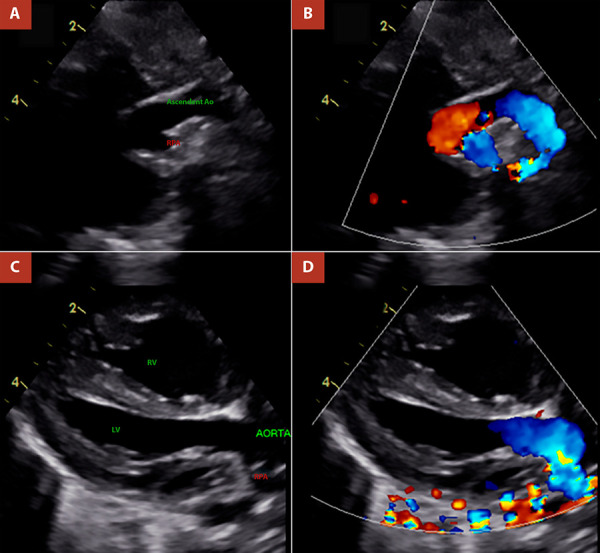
Transthoracic echocardiography, Case 02. A) and B) Suprasternal views showing the origin of the right pulmonary artery from the posterior wall of the ascending aorta. C) and D) Long-axis views demonstrating the origin of the right pulmonary artery from the ascending aorta. RPA: right pulmonary artery.
